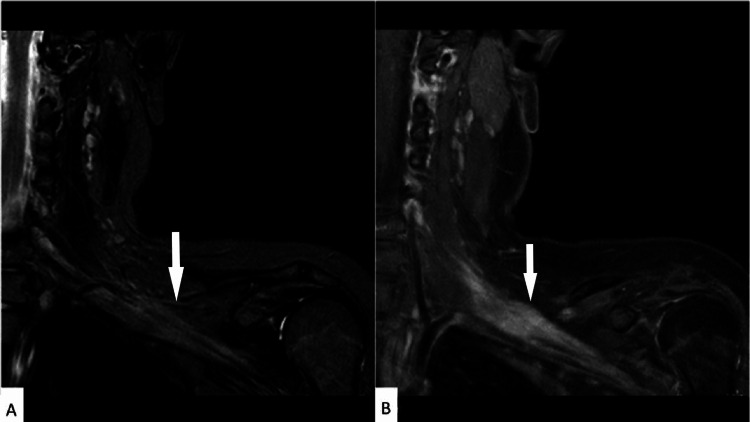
Magnetic resonance imaging of brachial plexus A) Coronal short-T1 inversion recovery (STIR) sequence demonstrates fusiform enlargement of the left middle and inferior trunks of the brachial plexus. The arrow points to an ill-defined thickening of the brachial plexus. B) Coronal T1 post-contrast with fat saturation sequence demonstrates corresponding enhancement. The arrow points to an ill-defined enhancement of the brachial plexus.
